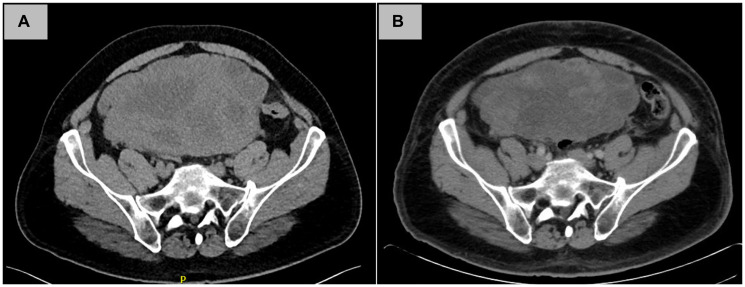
Radiographic tumor response to neoadjuvant imatinib therapy. (A) Contrast-enhanced computed tomography (CT) scan at initial presentation demonstrating a large heterogeneous mass occupying the lower abdomen and pelvis. (B) Repeat CT scan obtained after three months of imatinib therapy showing interval decrease in tumor size and internal complexity, consistent with partial radiographic response.
